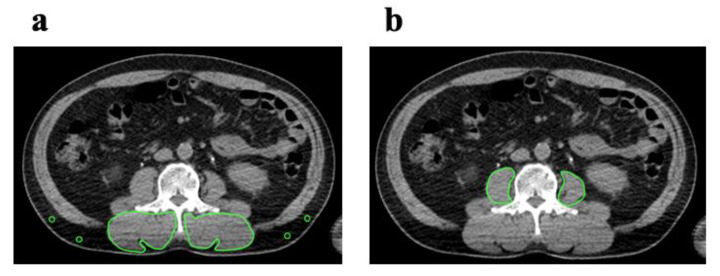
Cross-sectional CT images at the L3 level. (a) IMAC was evaluated by dividing the CT value of bilateral multifidus muscles according to the CT attenuation value for subcutaneous fat. (b) Subfascial muscle tissue in the multifidus muscle was precisely traced. The PMI was calculated by normalizing the cross-sectional areas to height (cm^2^/m^2^). CT, computed tomography; IMAC, intramuscular adipose tissue content; PMI, psoas muscle index.
